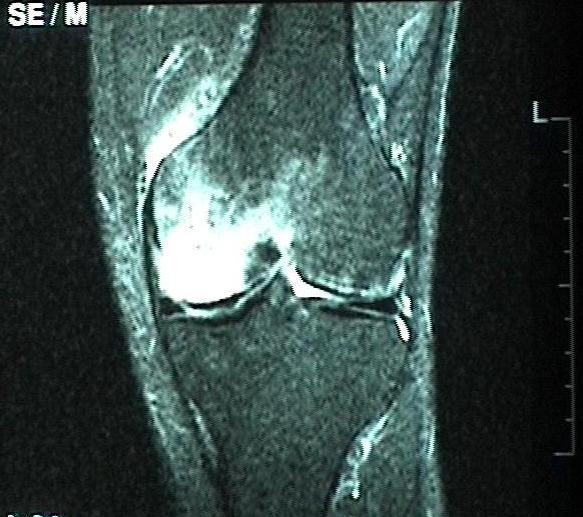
48-year-old man with a large BME in the medial femoral condyle not limited to the subchondral bone on the initial T2-weighted MR investigation (STIR).
